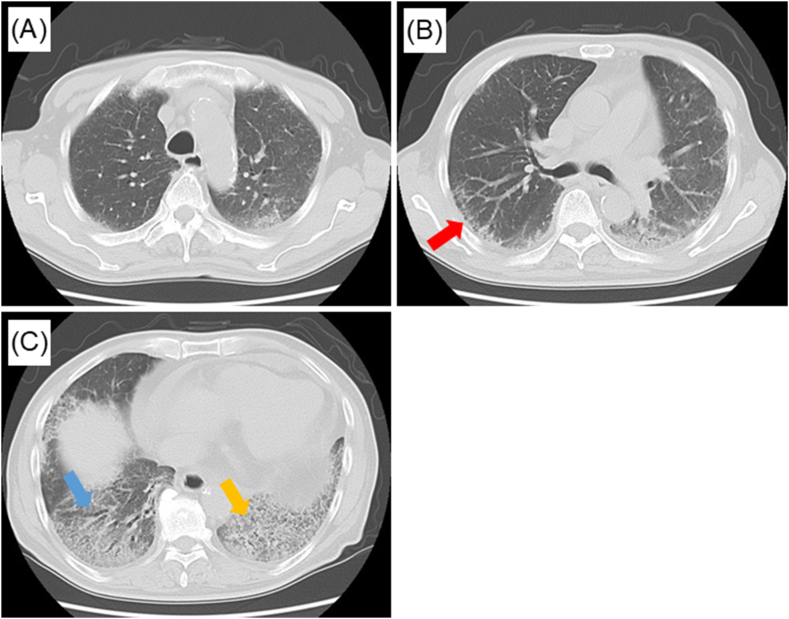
Chest computed tomography demonstrated subpleural reticular pattern (red arrow), traction bronchiectasis (blue arrow), and consolidation (yellow arrow) (A–C).
